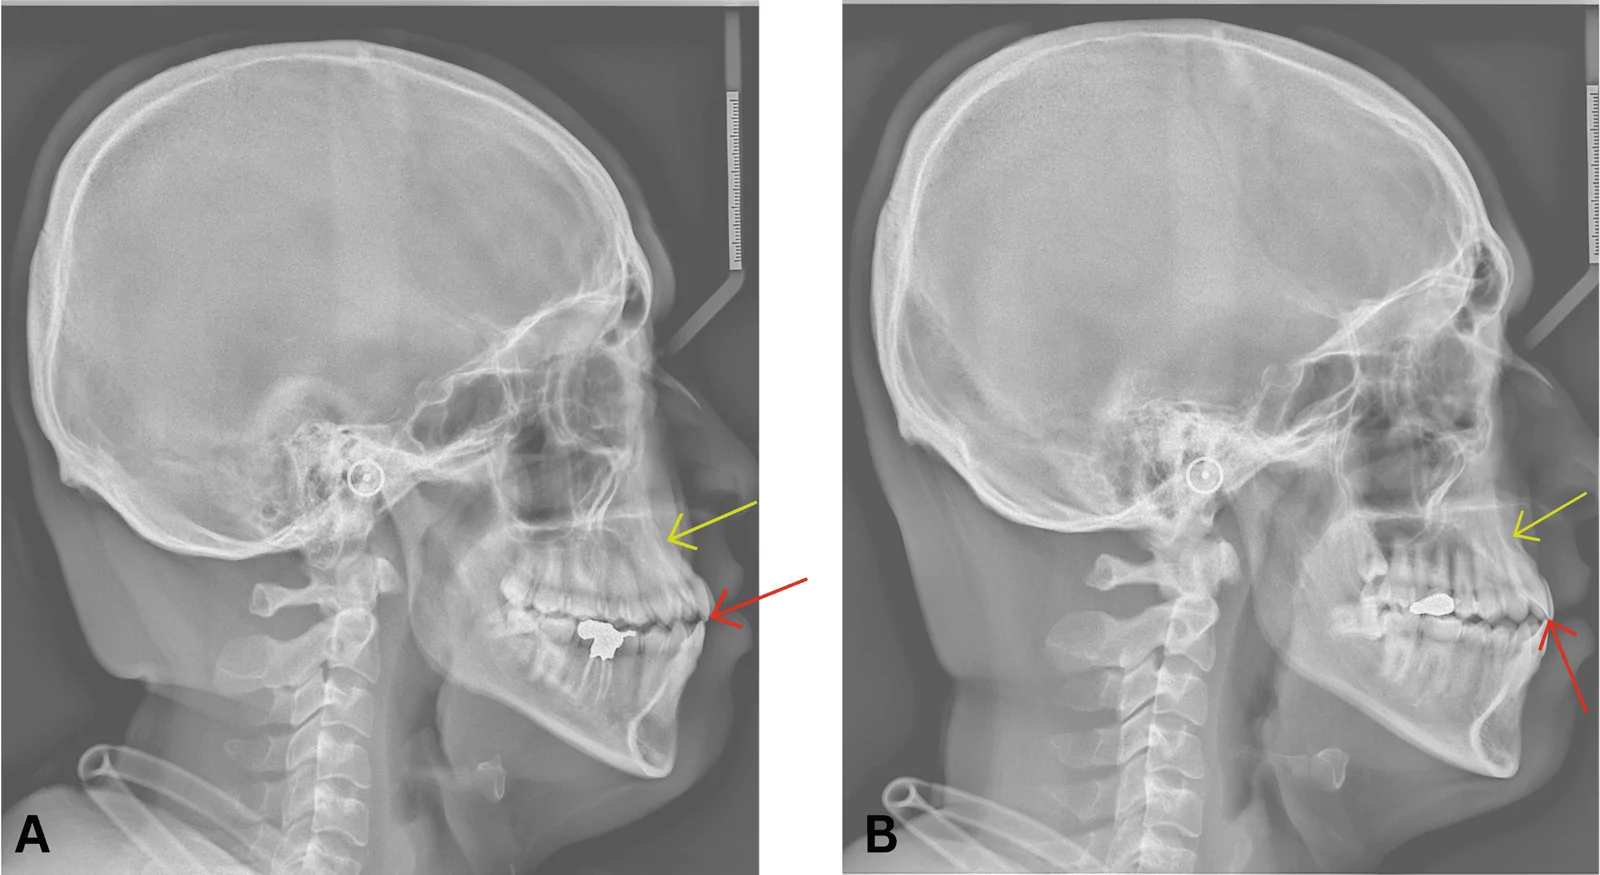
Lateral cephalometric radiographs of (A) T1 and (B) T2 The U1-SN angle differed between the twins, with T1 demonstrating retroclined maxillary incisors (101.67°) and T2 demonstrating normal maxillary incisor inclination (108.15°). The interincisal angle also differed between the twins: T1 had a normal interincisal angle (129.34°), whereas T2 exhibited a reduced interincisal angle consistent with more proclined incisors. T1: Twin 1, T2: Twin 2
